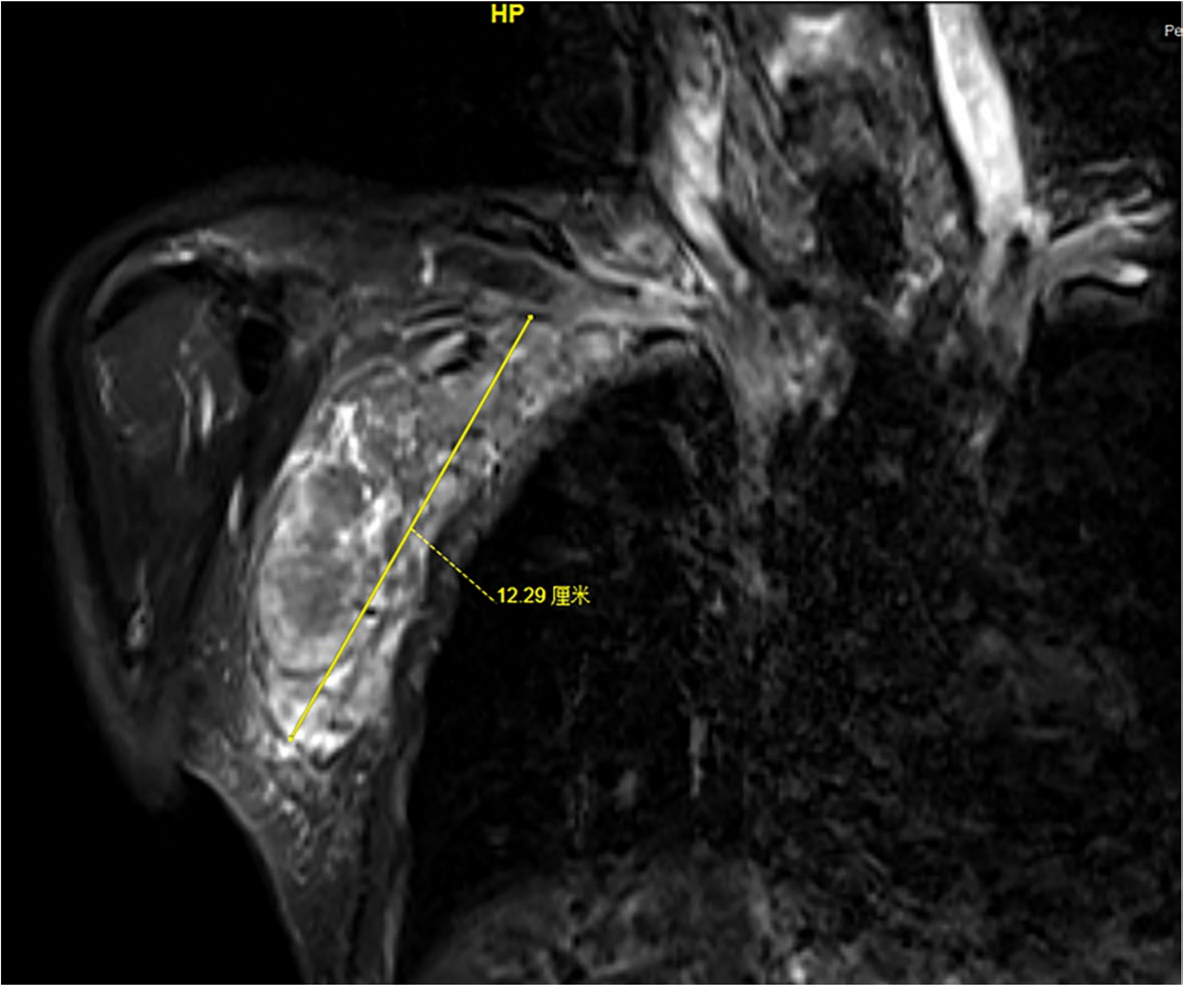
Manifestation of coronal scan of magnetic resonance imaging for the patient with right axillary fossa lymphatic metastasis before this combination treatment, who has progressed upon two lines of chemotherapy
